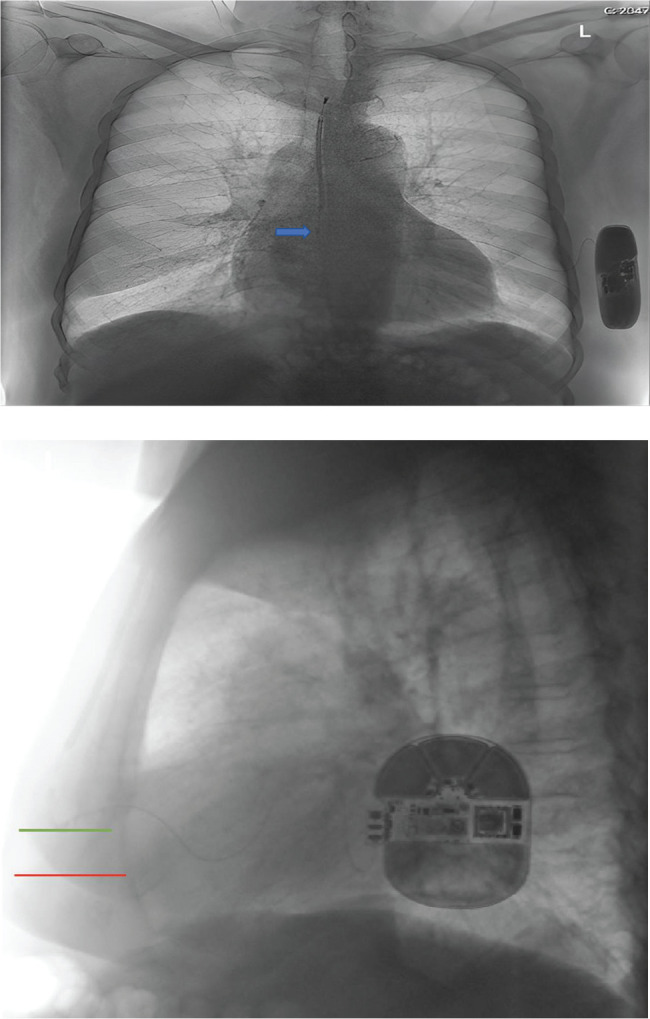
Chest X-ray (posteroanterior and lateral views). The blue arrow indicates the location of the ring electrode in the posteroanterior view. The red line indicates the tip of xiphisternum, and the green line indicates the xiphoid incision level at the appropriate location in the lateral view.
